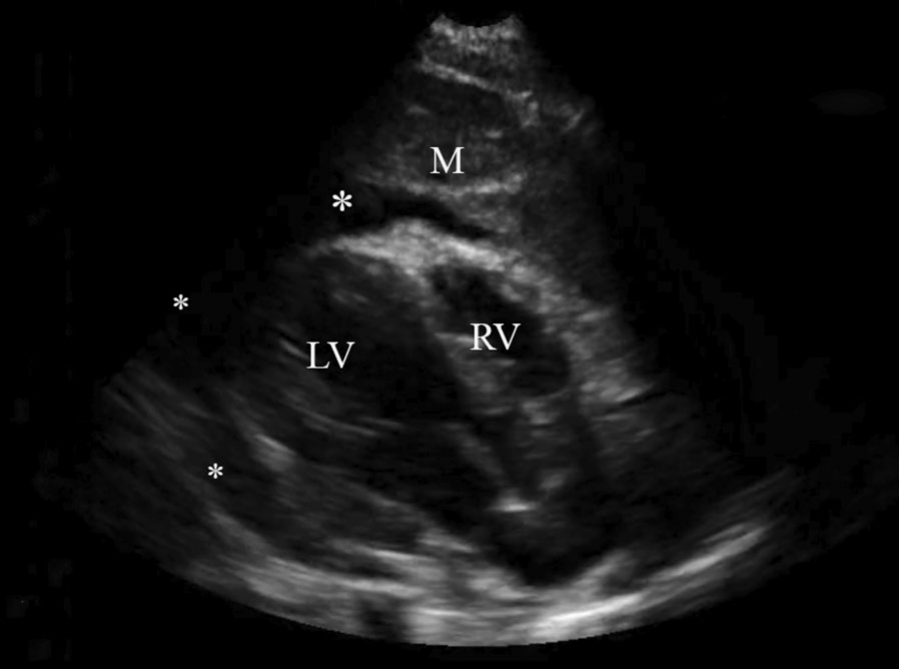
POCUS, Case 2. Parasternal long-axis view, revealing a circumferential pericardial effusion (*) and an anterior mediastinal mass (M). For orientation, the left ventricle (LV) and right ventricle (RV) can be visualized in this view
